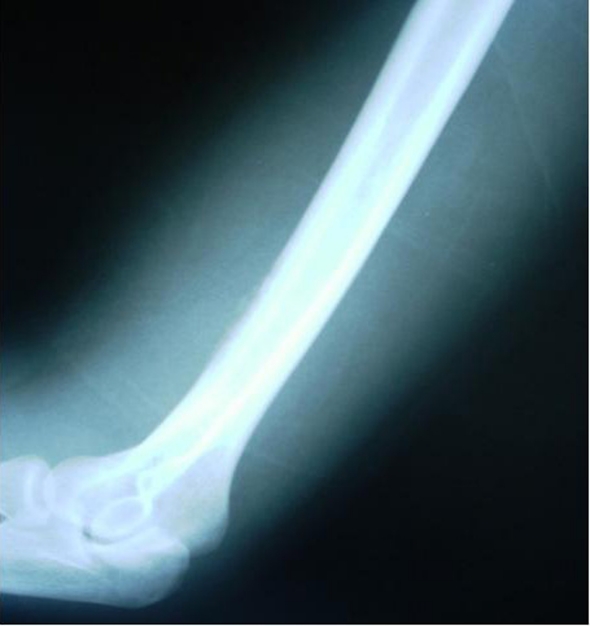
Lateral x-ray demonstrating the osseous reaction at the distal third of the humerus at the time of admission.
